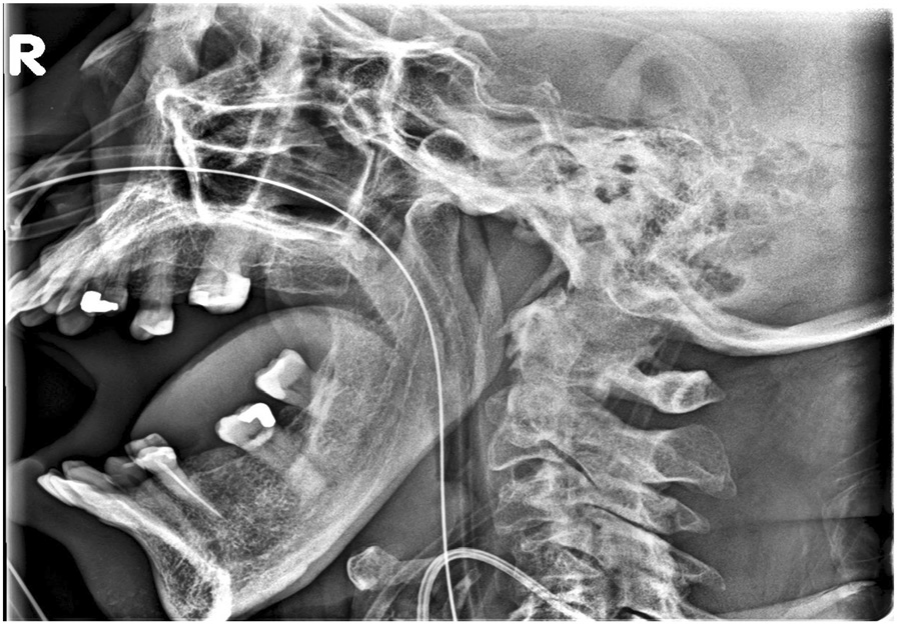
Lateral X-ray depicting anterior dislocation of the temporomandibular joint.
